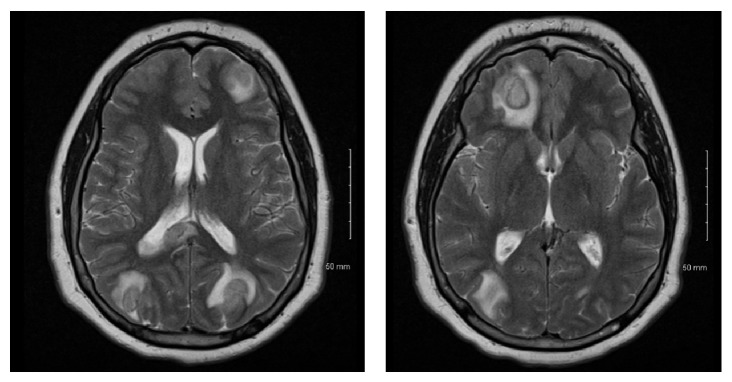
Brain MRI with contrast showing multiple ring-enhancing lesions and leptomeningeal enhancement.
